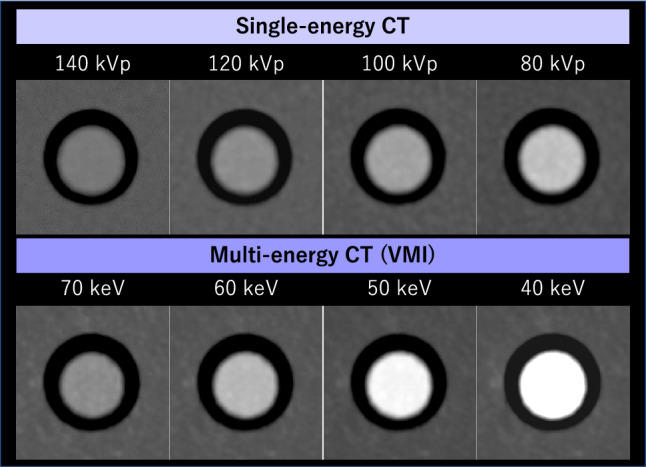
The iodine contrast of polyenergetic images ranging from 80 to 140 kVp in single-energy CT and VMI ranging from 40 to 70 keV in multi-energy CT (dual-layer detector scanner). VMI levels below 60 keV yield higher iodine contrast than polyenergetic 80 kVp images
